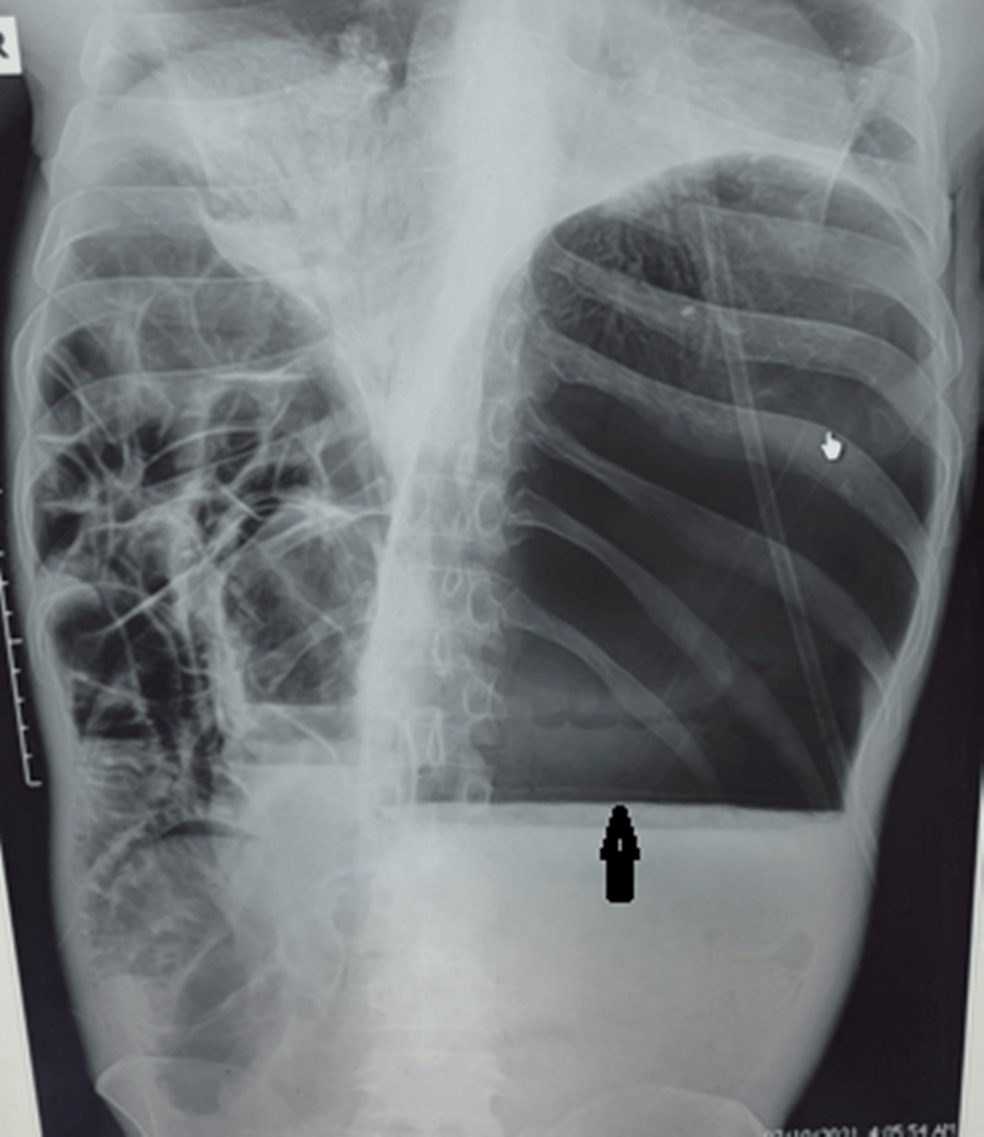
Erect X-ray of the abdomen showing severely distended stomach with fluid level (black arrow)
